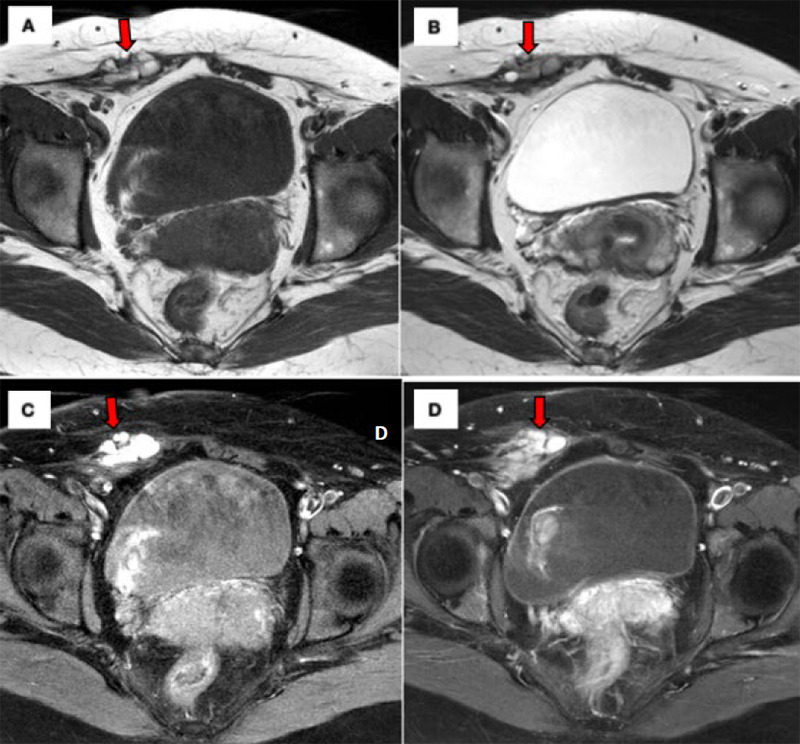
Magnetic resonance imaging of the pelvis in axial view: (A) Tl-weighted image depicts a lobulaten hyperintense lesion in the right rectn muscle. (1$) The lesion is heterogeneous and hypointense on die T2-weighted image, a characteristic known as ‘T2 shading’, and (C) hyperintense on the Tl fat-suppressed sequence, with no Oefinite enhancement in the poit-cnntrast study. (ID) The red arrow shows the right abdominal wall mass.
